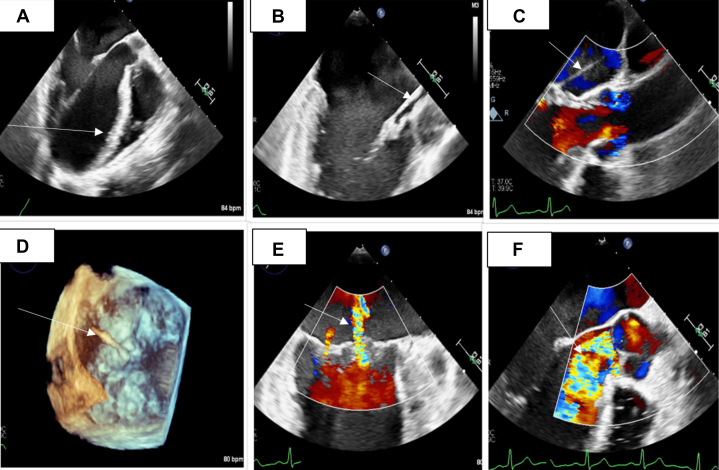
Transesophageal Echocardiogram The transesophageal echocardiogram demonstrates (A) an aberrant band of tissue (white arrow) attached to the anterior leaflet of the mitral valve at the A1/A2 region, (B, C) extending into the left atrium (white arrows). (D) The chord traverses the left ventricular outflow tract, passes through the aortic valve at the level of the left coronary cusp, and terminates in the ascending aorta (white arrow). (E) The mitral valve annulus appears dilated with mild-to-moderate mitral regurgitation (white arrow), showing both central and posterior jets. (F) Severe aortic regurgitation (white arrow) is also present, characterized by an eccentric jet and holodiastolic flow reversal in the descending thoracic aorta.
